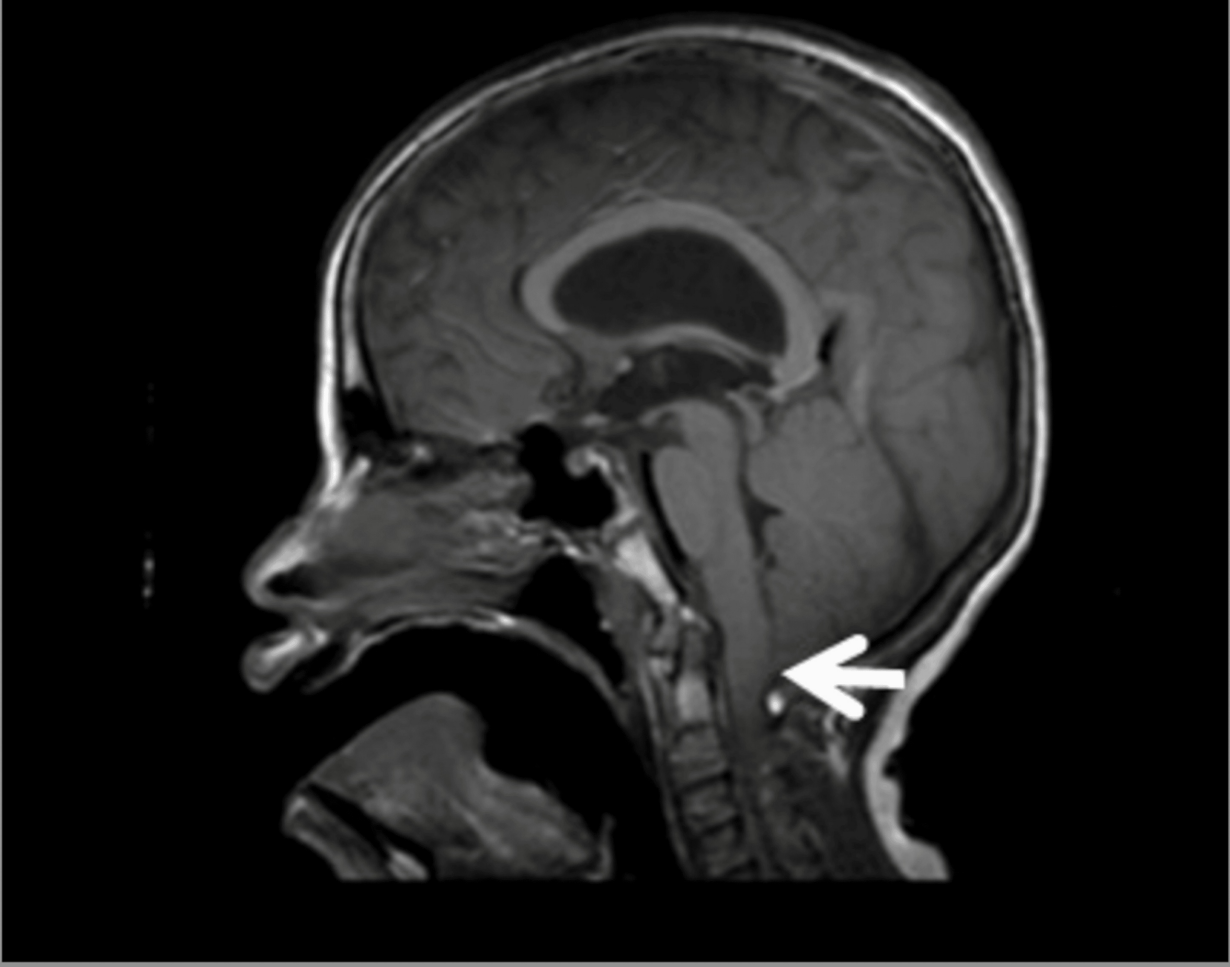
Sagittal MRI demonstrating cerebellar tonsillar herniation (arrow) Image reproduced from Open-i, National Library of Medicine (NIH) [21] (CC BY 3.0 Deed). MRI: magnetic resonance imaging
